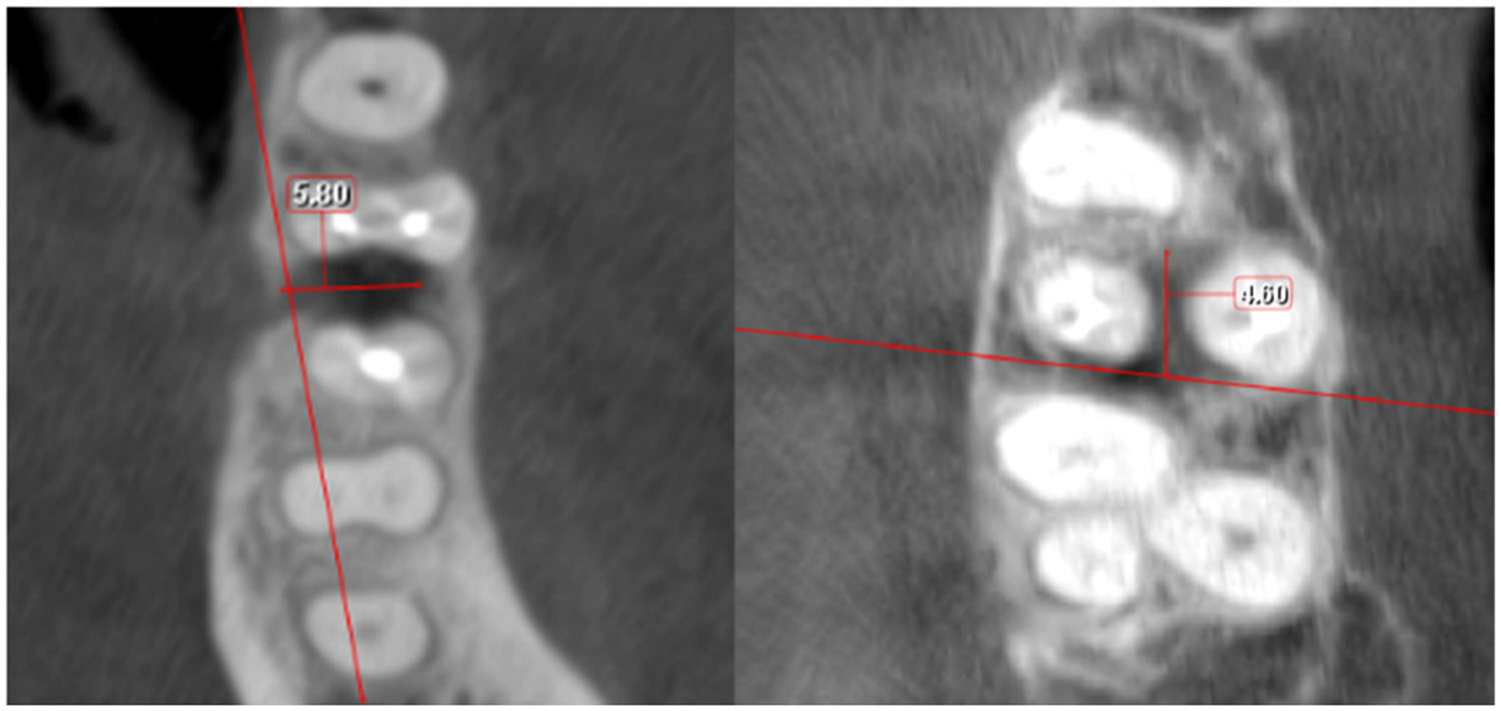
Radiographic assessment of the degree of furcation involvement.
